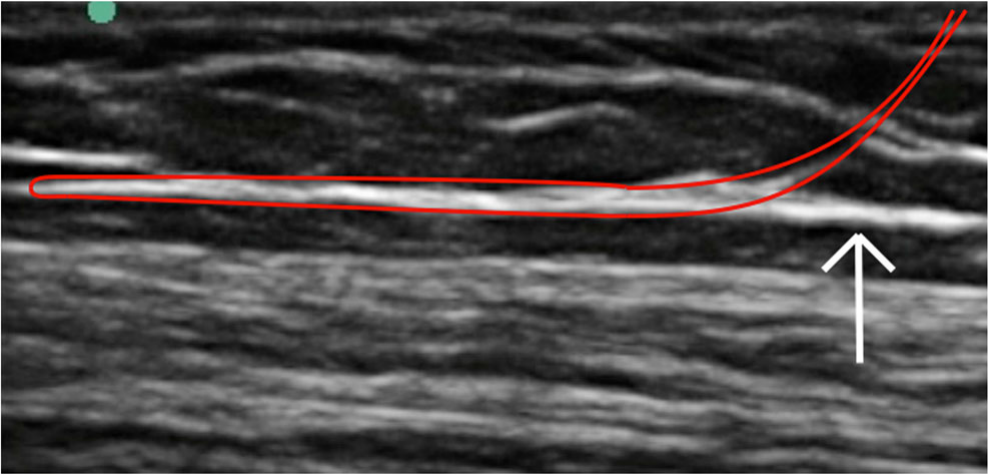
Ultrasound image demonstrating the intended location of the thread (*red line*), looped immediately superficial and deep to the fascial layer to be divided (*white arrow*)
